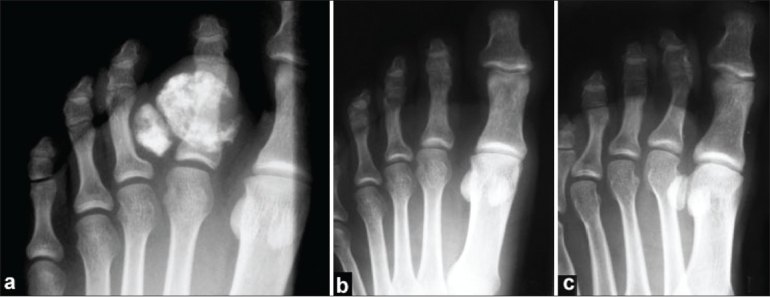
(a) Antero-posterior radiograph of the right foot showing two calcified lesions in relation to the proximal phalanx of the 2^nd^ toe. Radiograph of the right foot (b) anteroposterior view, and (c) oblique view, showing no recurrence at four years follow-up
